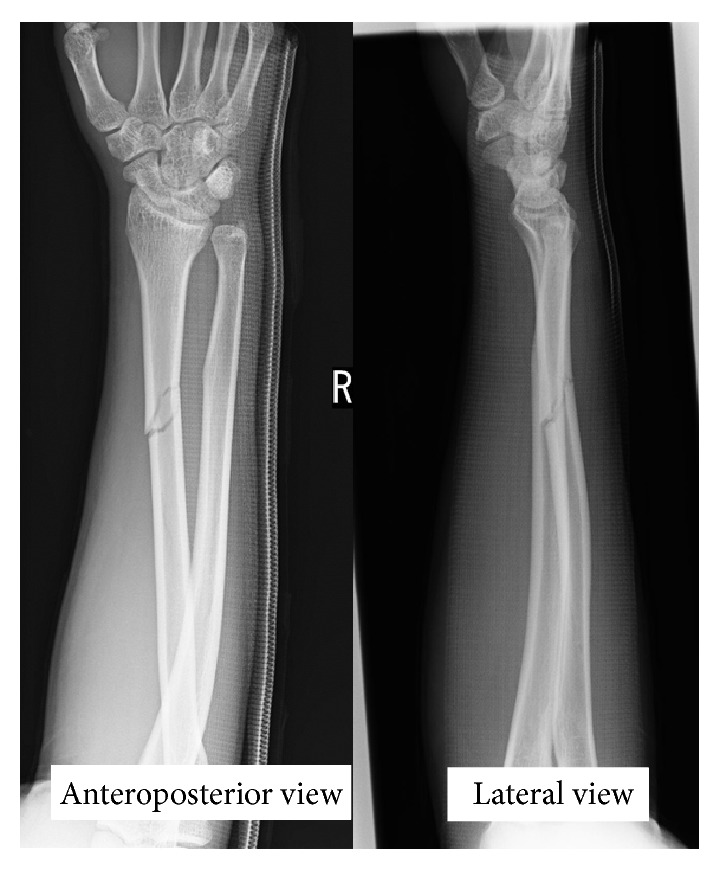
Radiograph of the fractured radius on admission.
